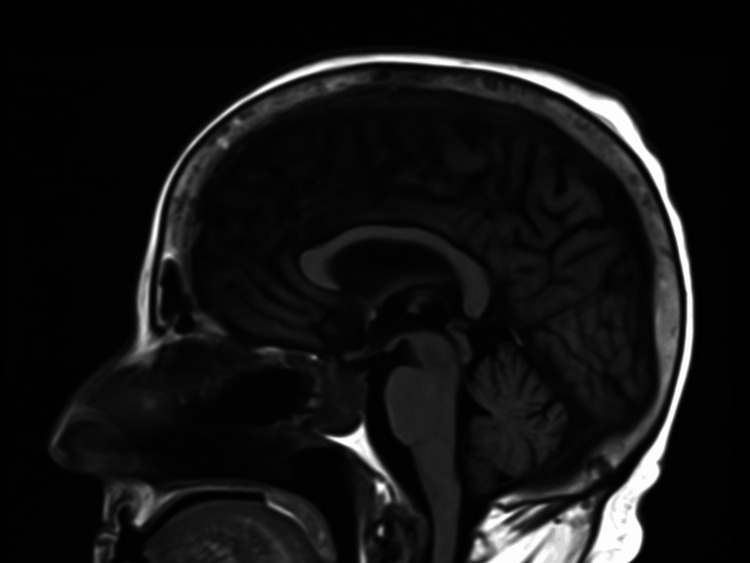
MRI of the brain (T1-weighted post-contrast sagittal image) obtained after the completion of one cycle of ICE chemotherapy and 26 fractions of craniospinal radiation, showing reduced mass effect on the optic chiasm with stable residual sellar disease and no evidence of progression. Interval imaging demonstrates improved decompression compared to baseline (Figure 1). ICE: ifosfamide, carboplatin, and etoposide; MRI: magnetic resonance imaging
